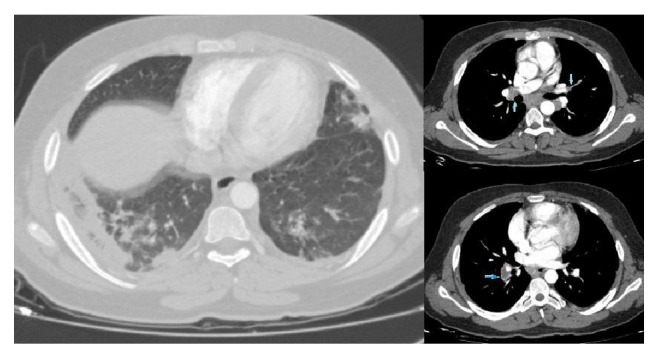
PTE was detected on the right main pulmonary artery and left pulmonary artery branches and consolidation areas in bilateral lower lobe and areas of ground glass in the upper and lower lobes in computed tomography pulmonary angiography.
